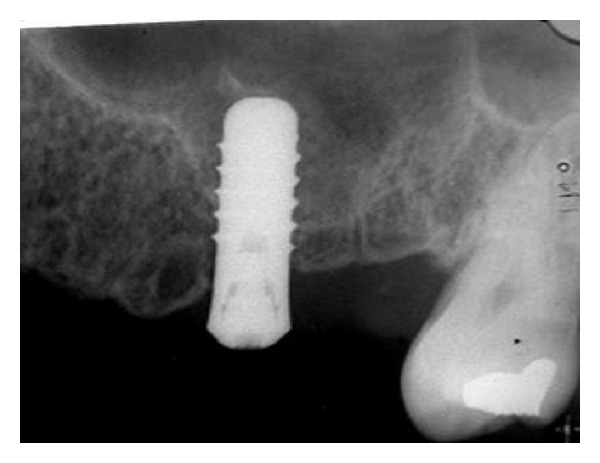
Radiograph taken after completion of the OSFE procedure without bone grafting with 8 mm lifting of the sinus membrane and a residual bone height of less than 3.0 mm and the amount of sinus floor elevation.
